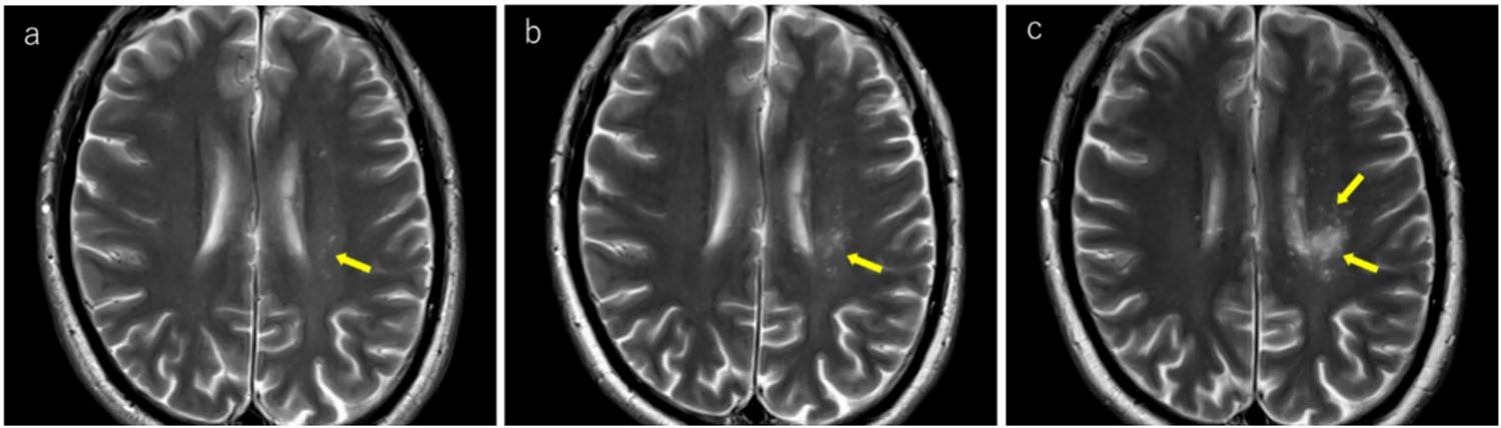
Same patient as in Fig. 2: an HIV-positive man with PML in his 30 s. a T2-weighted image showing the emergence of a punctate pattern in the deep white matter around the left lateral ventricle (yellow arrow). b One month later, the lesions show local expansion (yellow arrow). c After an additional month, the lesions showed further local expansion and milky way appearance (yellow arrows)
